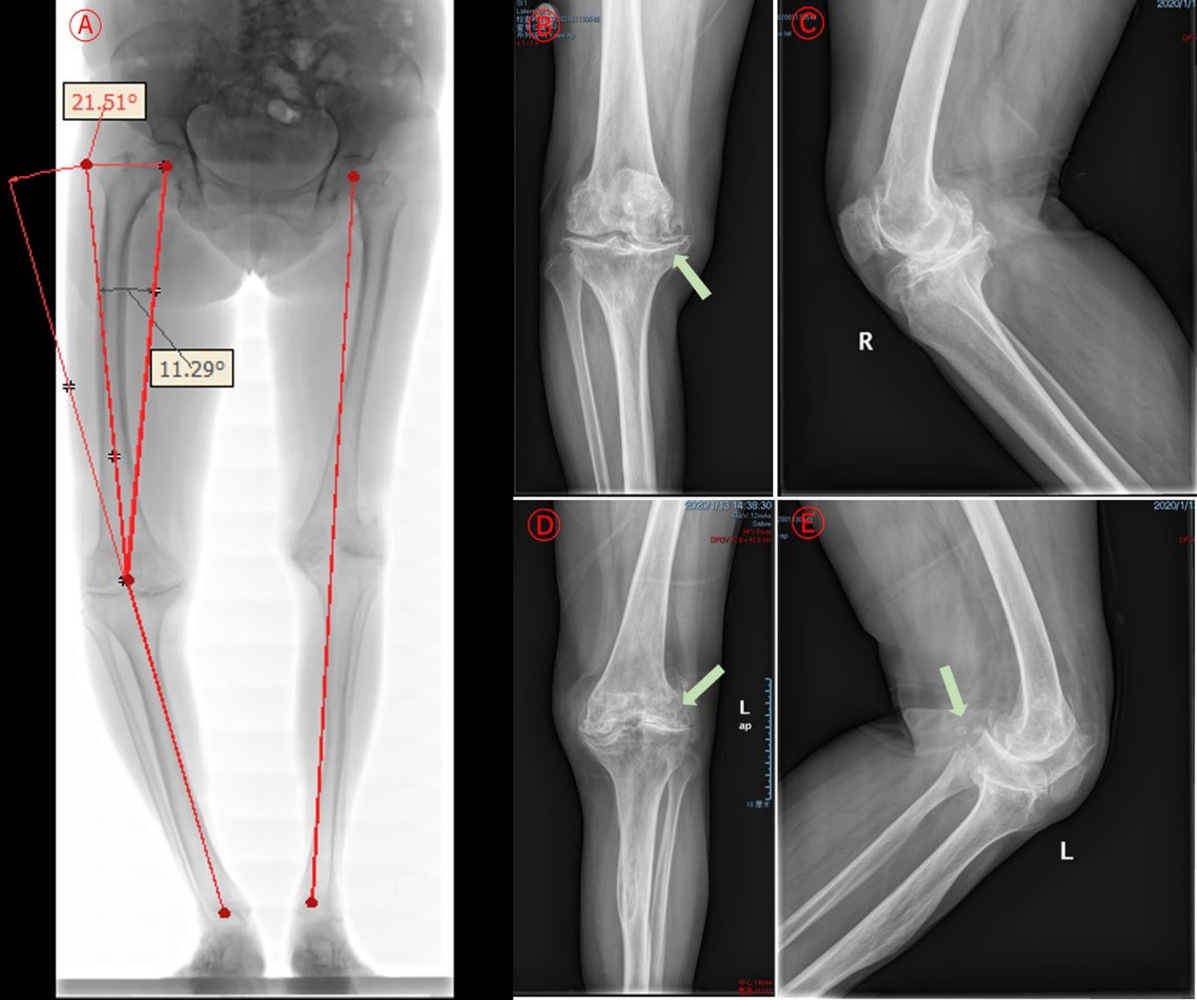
Characteristics of bilateral lower limb and knee DR at admission. a Long-leg radiograph showed bilateral femur and tibia dysplasia with deformation of the shafts. The right varus angulation was 21.5°, and the FMAA was 11.3°. The mechanical axis of the left lower limb was normal. b–e Bilateral knee joint AP & LAT showed that the extensive subchondral bones of the knees were sclerotic, and the articular surfaces were out of flatness. Ambilateral joint gaps were significantly narrowed, especially in the lateral left knee and medial right knee. A couple of loose bodies were seen in the left knee
